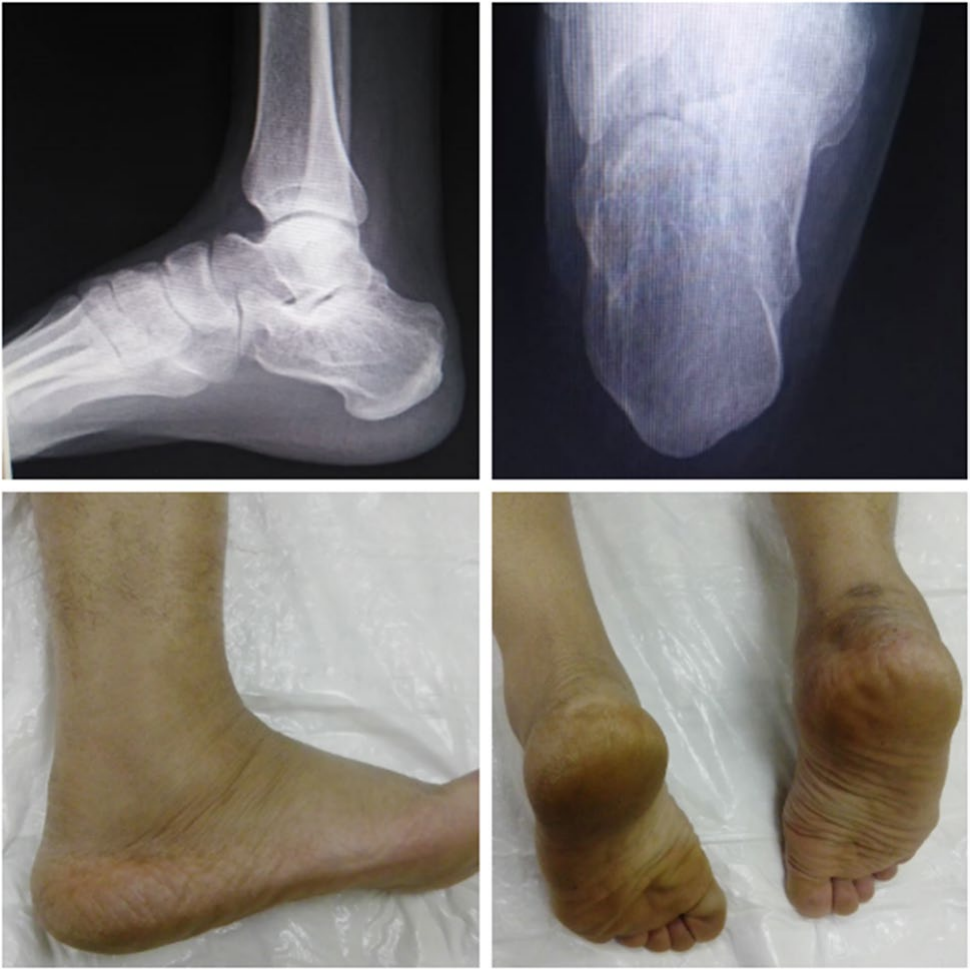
Final radiographic and clinical follow-up of patient with K-wire fixation
